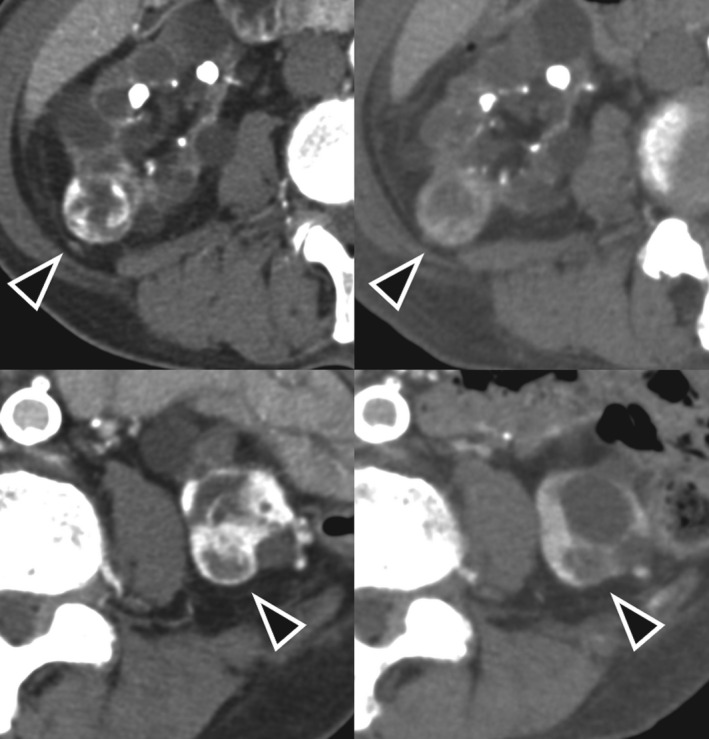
Contrast‐enhanced CT of the renal tumors before combination therapy with ipilimumab plus nivolumab (upper left: right kidney tumor; lower left: left kidney tumor) and after 6 months of treatment (upper right: right kidney tumor; lower left: left kidney tumor). No remarkable changes are observed
